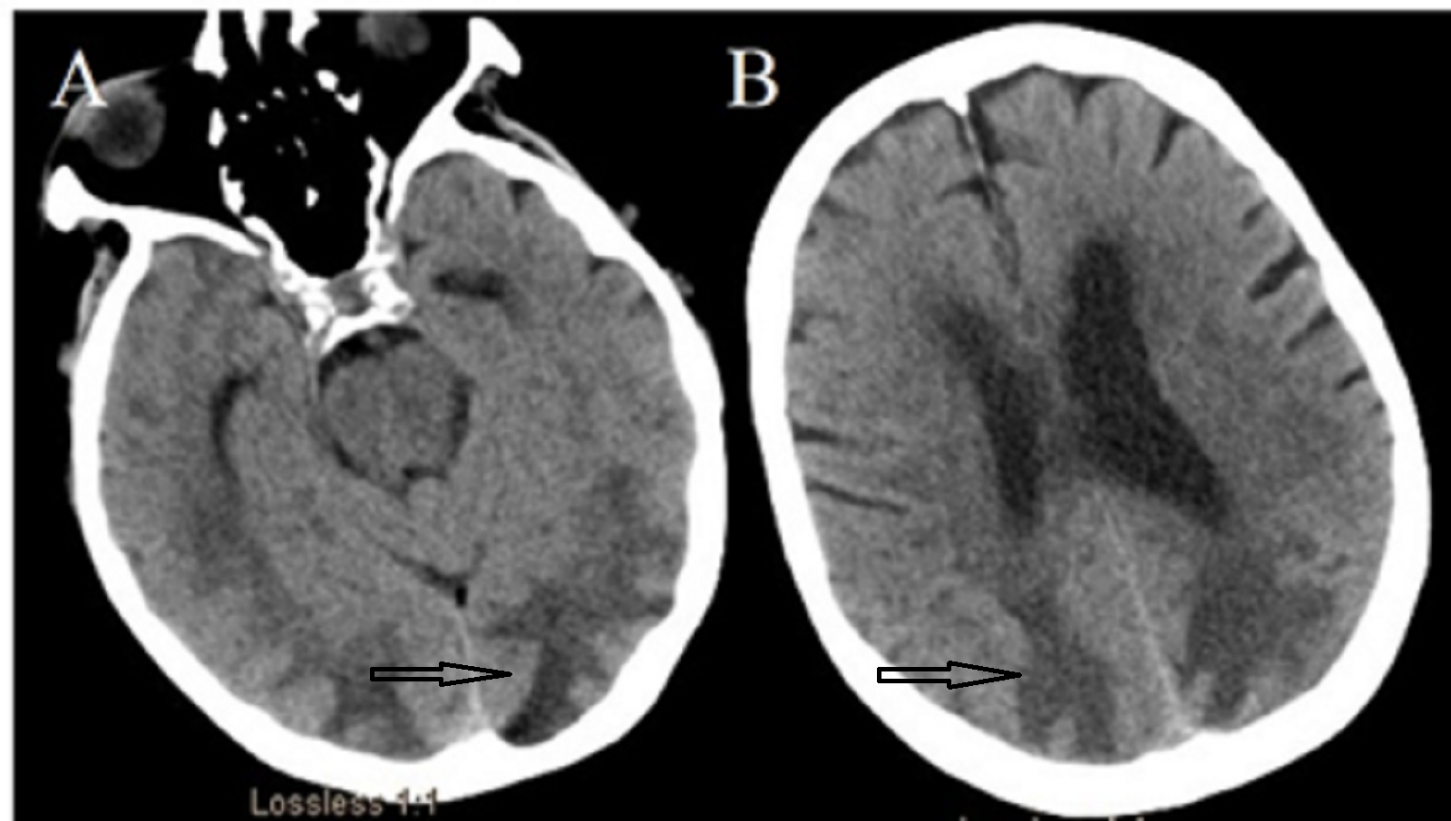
Computed tomography (CT) of the head without contrast CT showing bilateral posterior quadrant hypodensities consistent with vasogenic edema. These are typical radiological findings in posterior reversible encephalopathy syndrome (PRES).
